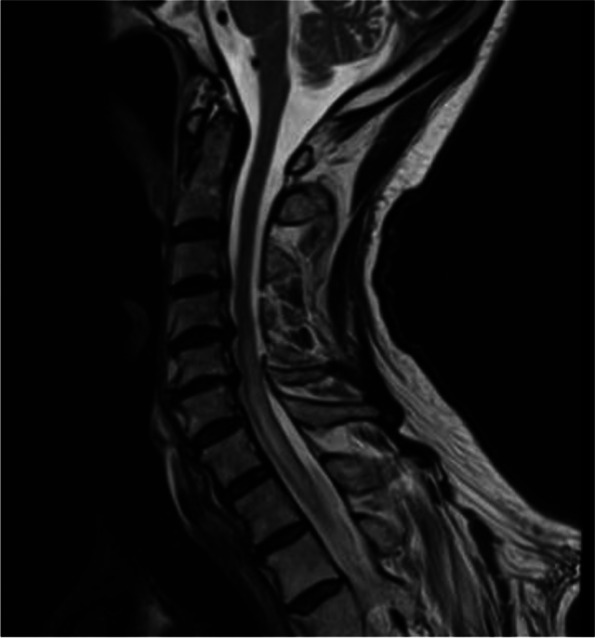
MRI cervical spine: sagittal T2: hyper intense intra-medullary T2 signal
